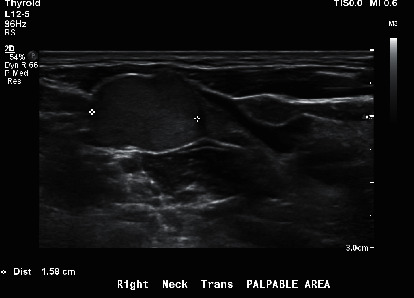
Ultrasound transverse image of the right palpable swelling showing abnormal cervical lymph node with localized invasion.
